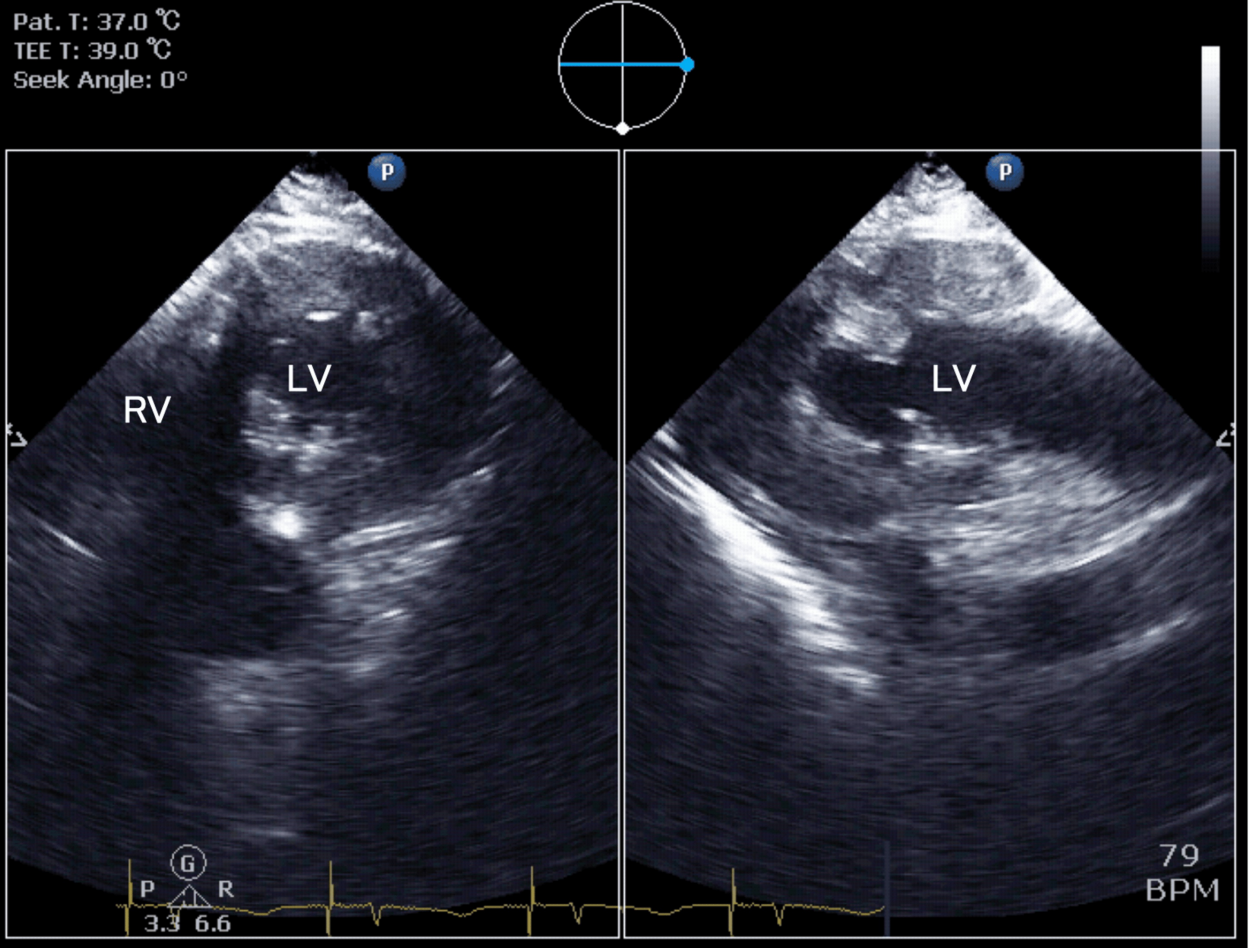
Post-procedural transesophageal echocardiography, transgastric bi-plane left ventricular view This image simultaneously bi-plane displays the short-axis and long-axis views of the left ventricle after tumor resection and weaning from cardiopulmonary bypass. The D-shaped deformity of the left ventricle that was observed preoperatively has improved. RV, right ventricle; LV, left ventricle
